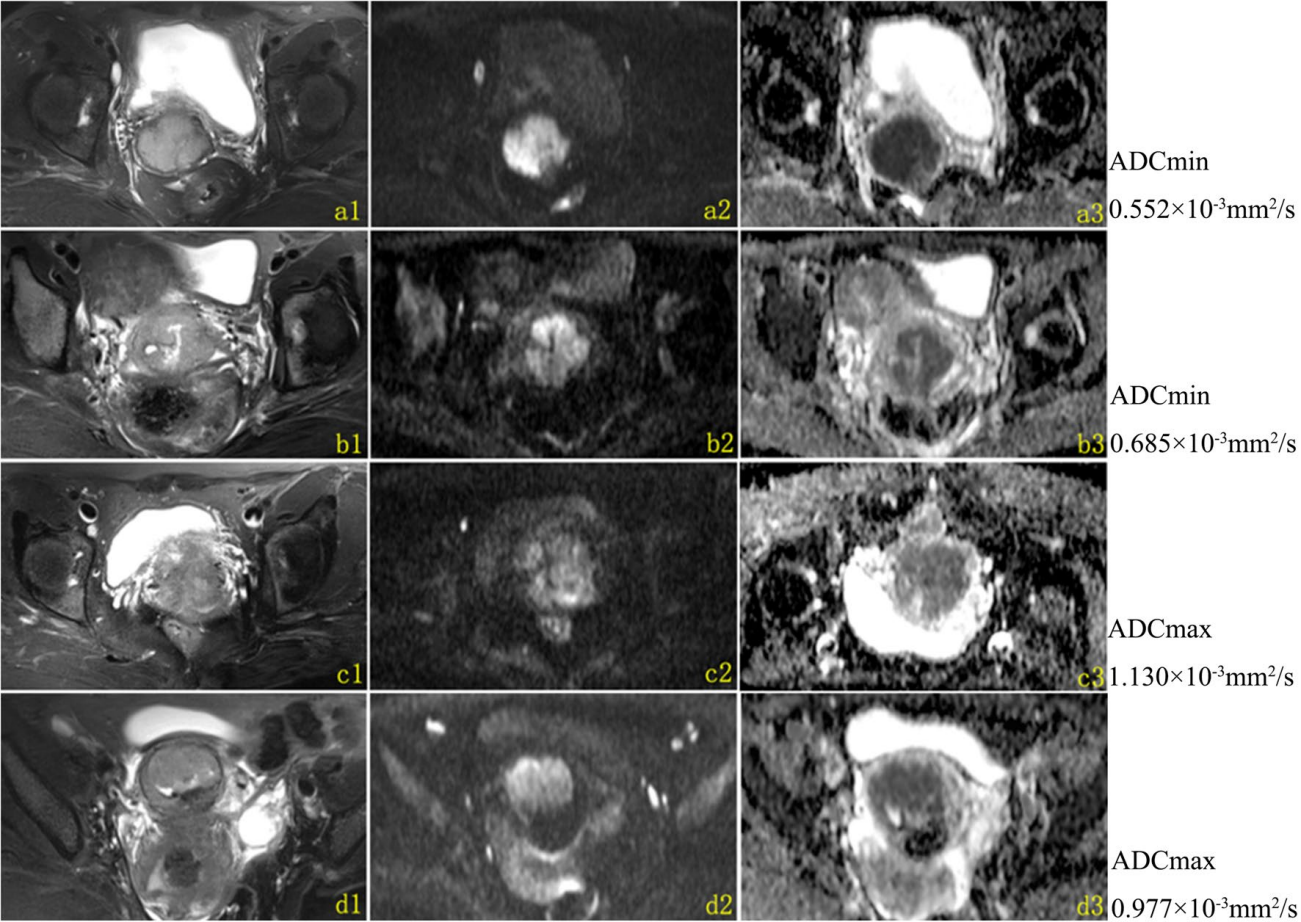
Different ADCmax and ADCmin values of lesions treated with NAT + RH (a–b) and CCRT (c–d); the maximum slice of the primary tumor was showed on T2W(a1-d1), DWI(a2-d2) and ADC(a3-d3) map. a1–3 A 51-year-old patient treated with NAT + RH, the ADCmin of the primary tumor was 0.552 × 10^−3^mm^2^/s, DFS was 11.23 months; b1–3 a 45-year-old patient treated with NAT + RH, the ADCmin of the primary tumor was 0.685 × 10^−3^mm^2^/s, DFS was 68.90 months; c1–3 a 48-year-old patient treated with CCRT, the ADCmax of the primary tumor was 1.130 × 10^−3^mm^2^/s, DFS was 10.70 months; d1–3 a 48-year-old patient treated with CCRT, the ADCmax of the primary tumor was 0.977 × 10^−3^mm^2^/s, DFS was 35.50 months. NAT + RH, neoadjuvant therapy followed by radical hysterectomy; CCRT, concurrent chemoradiation; DFS, disease-free survival
